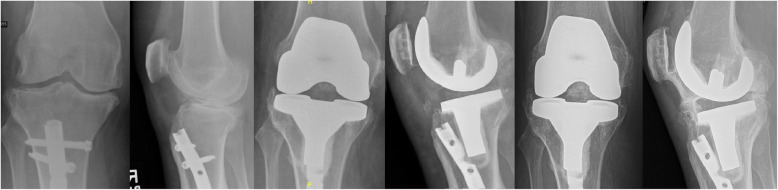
Preoperative, postoperative, and final follow-up radiographs of a patient who sustained joint ankylosis after conversion total knee arthroplasty (TKA) with partial intramedullary nail (IMN) removal
